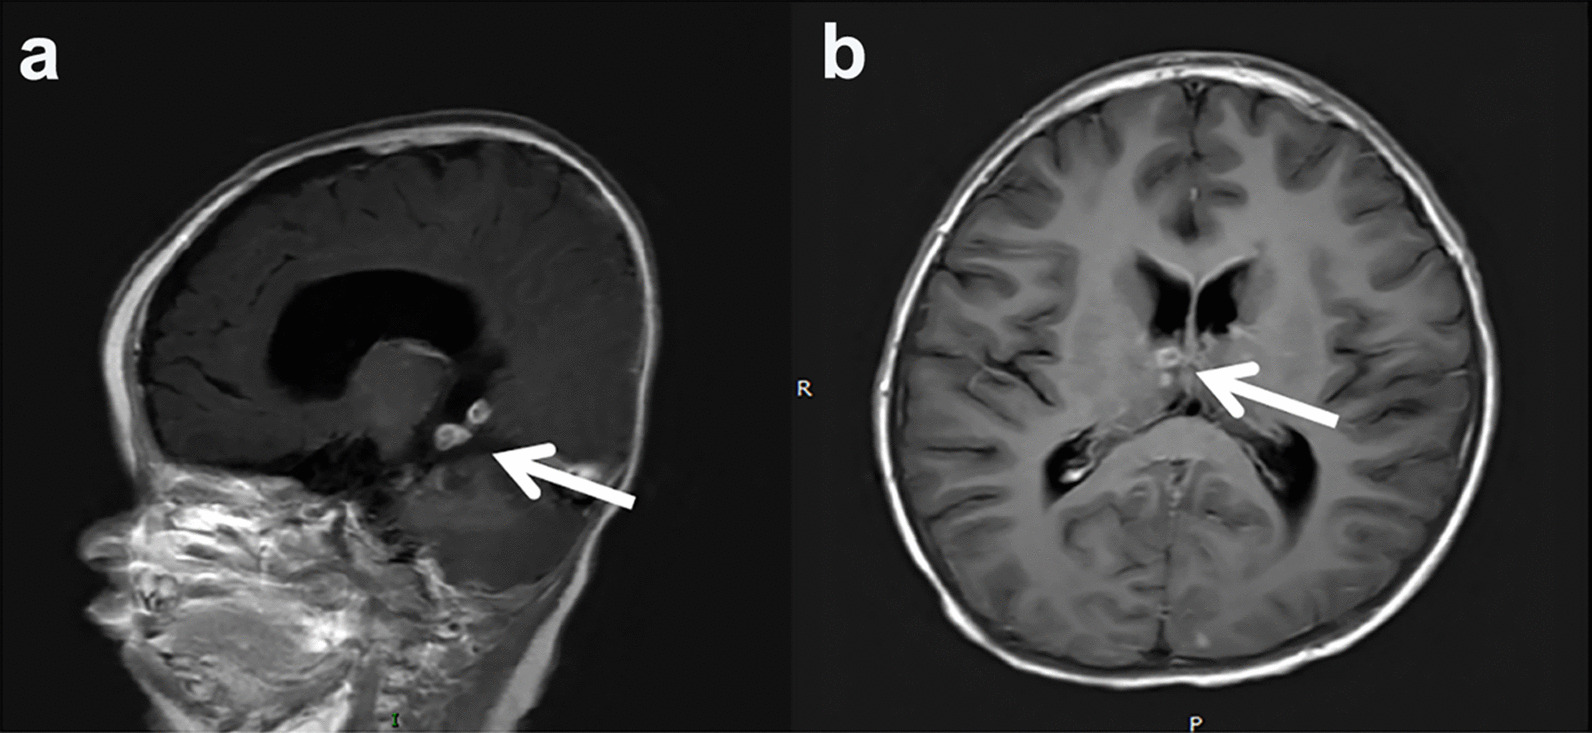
The typical granuloma changes of 2 CNSC cases according to enhanced brain MRI. a T1WI, sagittal scans; b T1WI, coronal scans. *CNSC* central nervous system candidiasis due to *Candida albicans*
